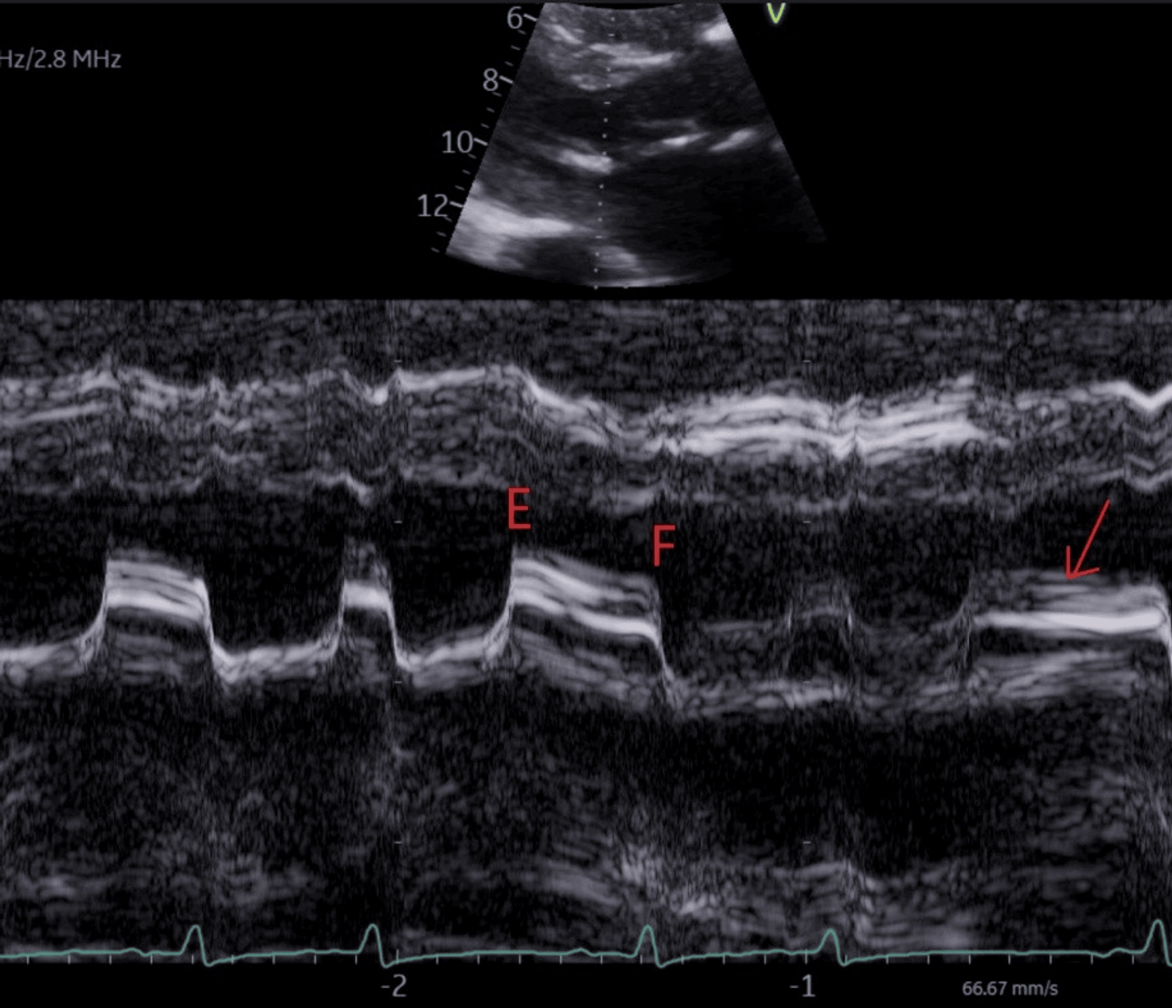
Transthoracic echocardiography, M-mode, showing thickened mitral valve leaflets (arrow) with reduced E-F slope of the anterior mitral leaflet and absent mitral valve A-wave.
